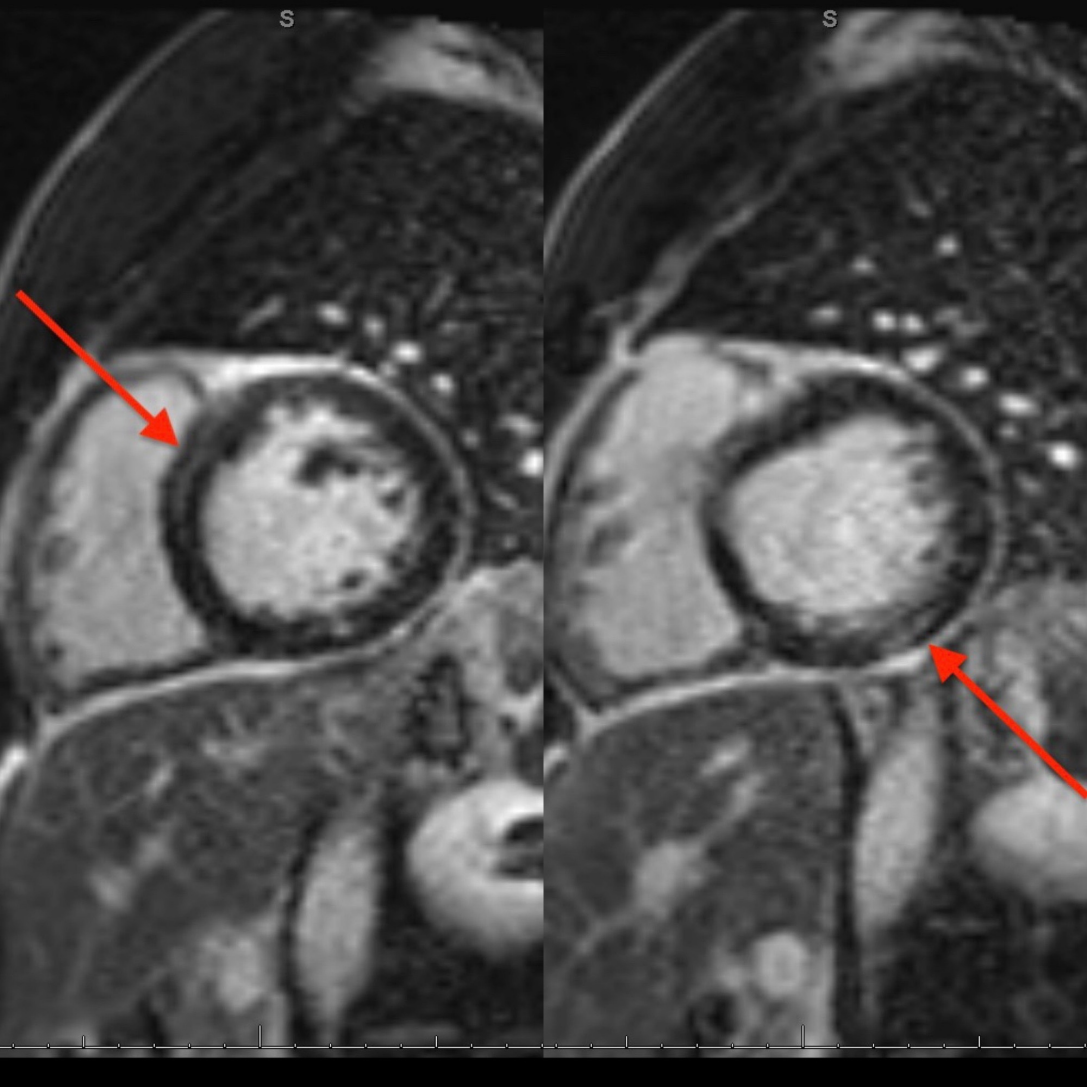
Cardiac magnetic resonance imaging (MRI) showing early gadolinium enhancement (left arrow) and a pattern of patchy mid-myocardial delayed enhancement (right arrow).
